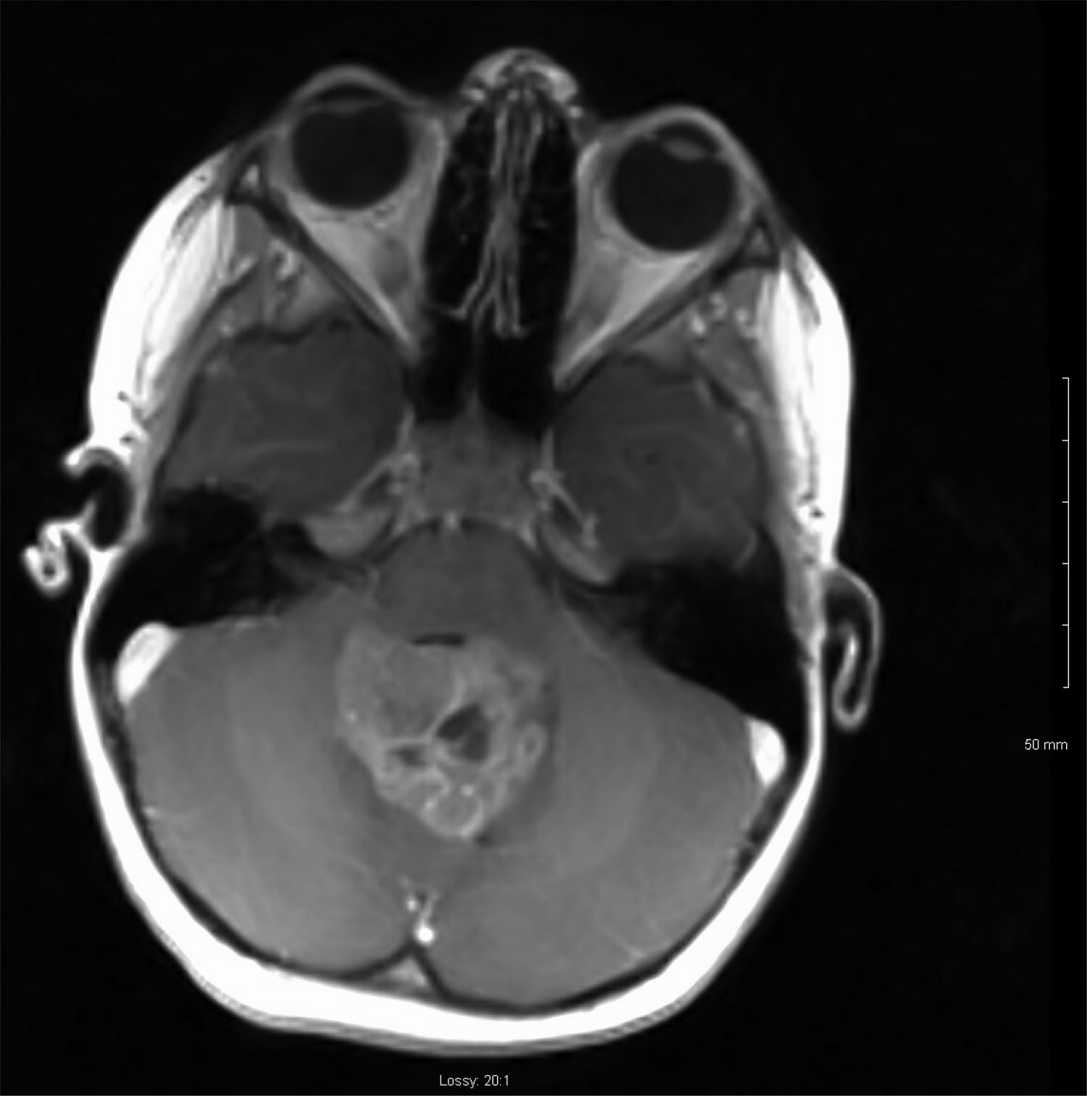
Preoperative MRI brain (axial view) of fourth ventricle tumour
